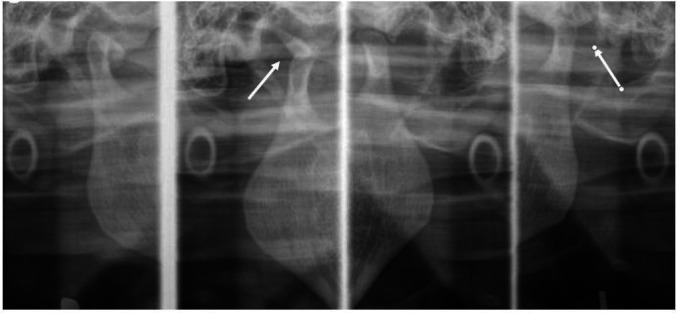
Panoramic X-ray showing Loose bodies on the right and left side of TMJ (arrows).
